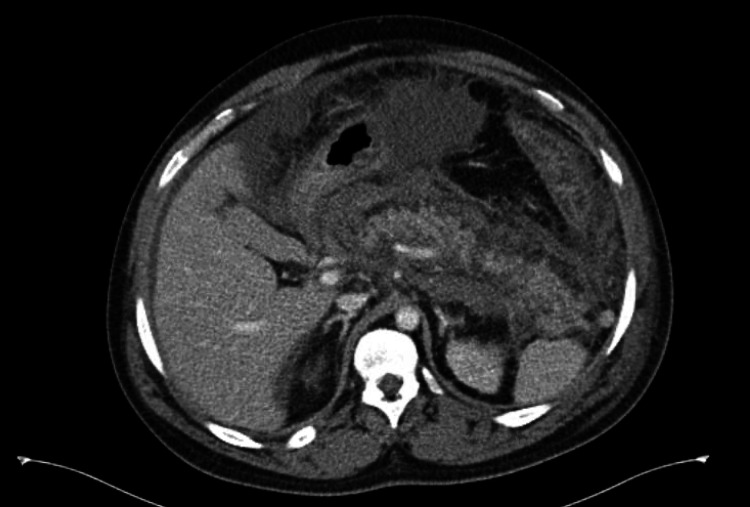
Repeated CT scan of the abdomen CT scan of the abdomen with IV contrast demonstrated necrotizing hemorrhagic pancreatitis, progression of intraabdominal edema, and hemoperitoneum CT: computed tomography
